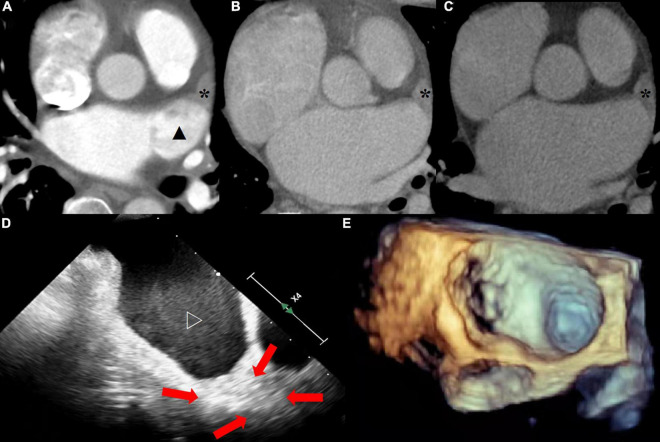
(A) The arterial enhanced phase shows the filling defect (solid star) at the tip of the left atrial appendage and the uneven filling (solid triangle) of contrast medium in the rest of the structure. (B) The tip of left atrial appendage still showed filling defect, while the rest were filled evenly at 1-min delayed. (C) Thrombus at the tip of the left atrial appendage was confirmed at 3-min delayed. (D) The tip of left atrial appendage was old thrombus (red arrow) and the rest of the structure were severe SEC (hollow triangle) at TEE. (E) Image of 3D ultrasound.
